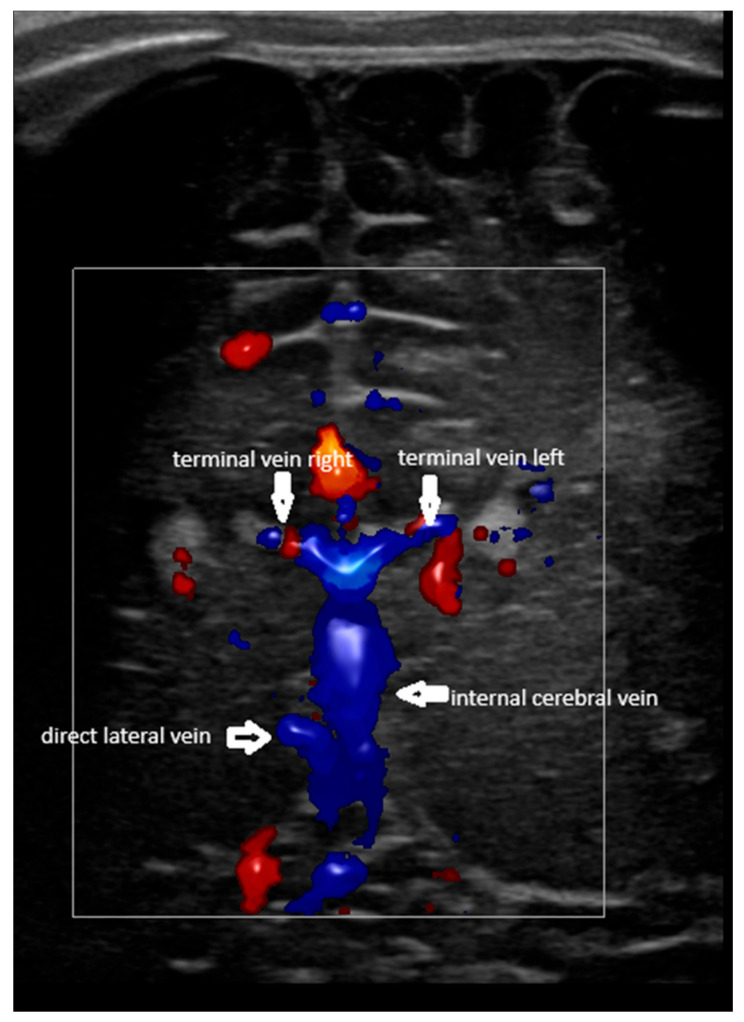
Coronal section; plan slightly posterior to the foramen of Monro. Colour Doppler. Normal pattern; confluence between the terminal (thalamostriate) veins and the internal cerebral vein identified. The direct lateral vein on the right is also visible on the right. The confluence is, in this case, slightly posterior to the foramen of Monro.
